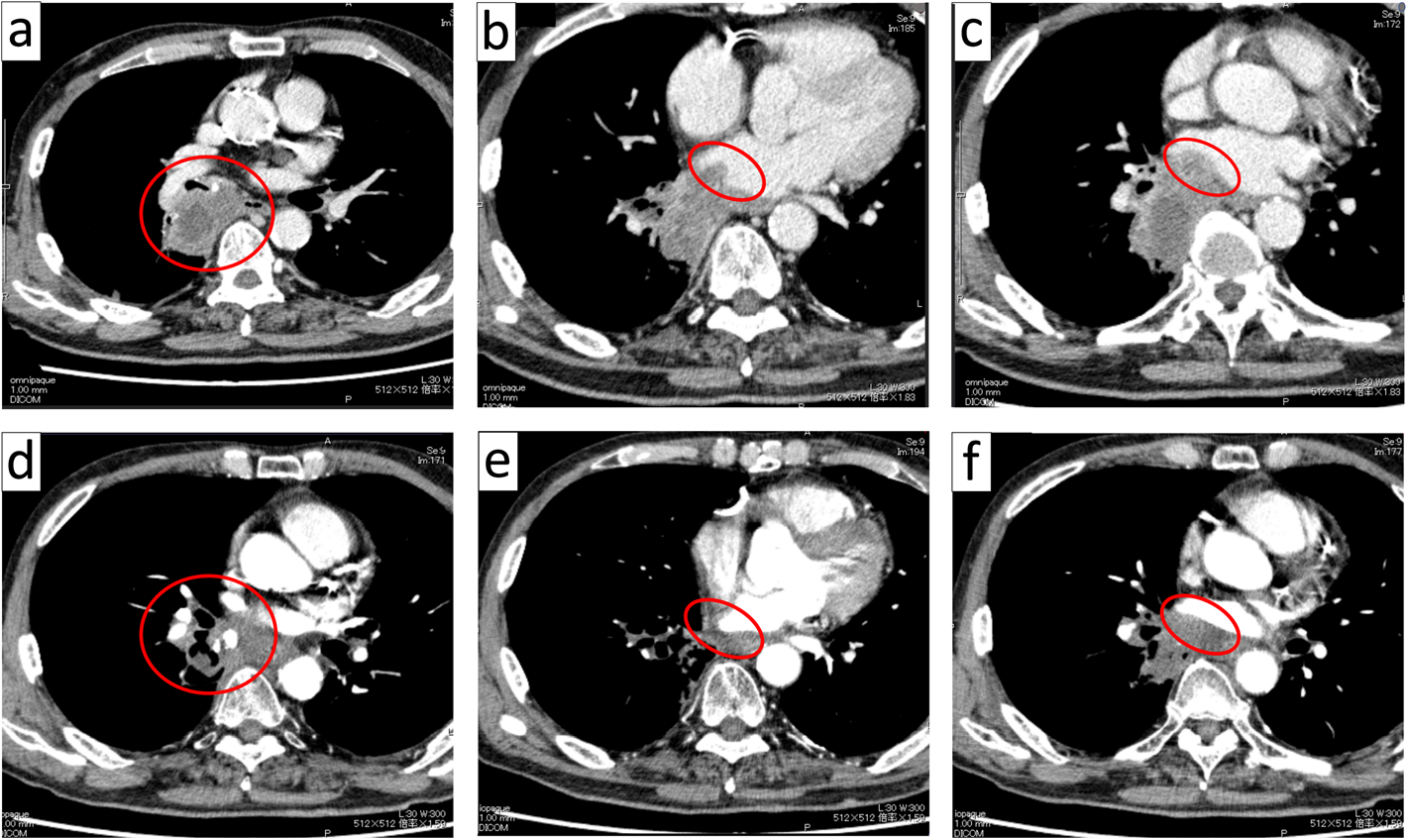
Chest computed tomography (CT) before chemoradiotherapy (CRT) (a–c) and before surgery (d–f)
